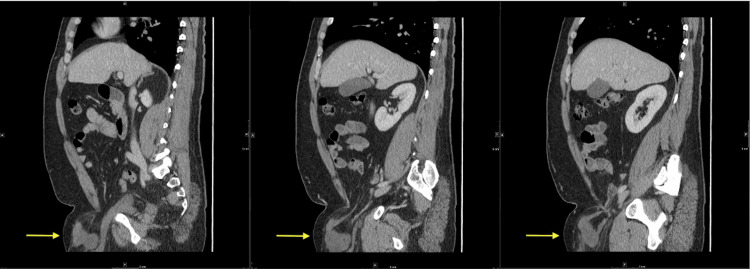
Sagittal CT series of bladder and small bowel segment traversing abdominal wall defect into the hernia sac.
